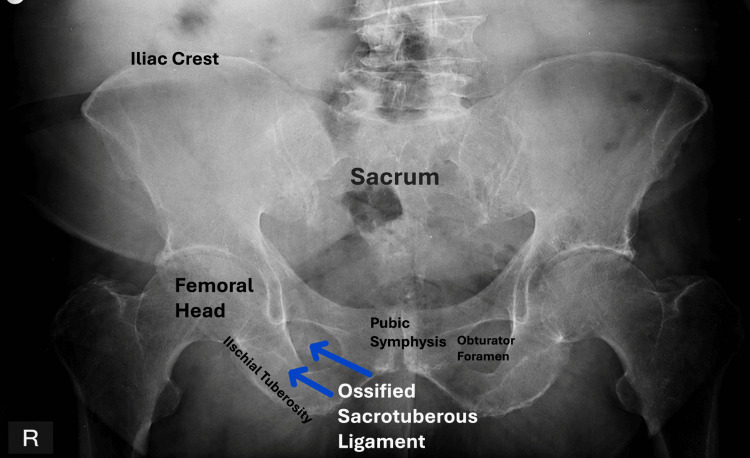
Anteroposterior pelvic radiograph Partial ossification of the right sacrotuberous ligament is demonstrated (arrows), appearing as a fan-shaped ossified structure within the right posterior pelvis adjacent to the ischiopubic ramus. Key anatomical landmarks demonstrated include the sacrum, right iliac crest, right femoral head, right ischial tuberosity, left obturator foramen, and pubic symphysis.
